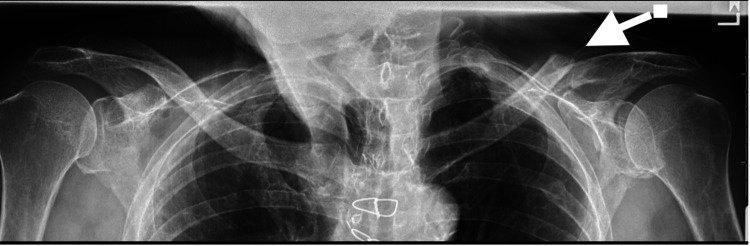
One-year postoperative radiograph anteroposterior view showing even alignment of both clavicles with no residual superior displacement of the left medial clavicular fragment
